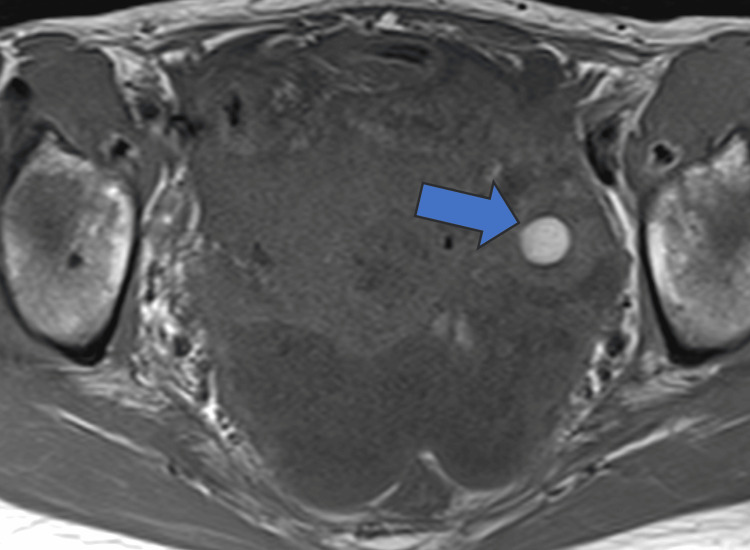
Gadolinium contrast-enhanced MRI scan (horizontal section) Hemorrhagic cyst of the left ovary (arrow).
